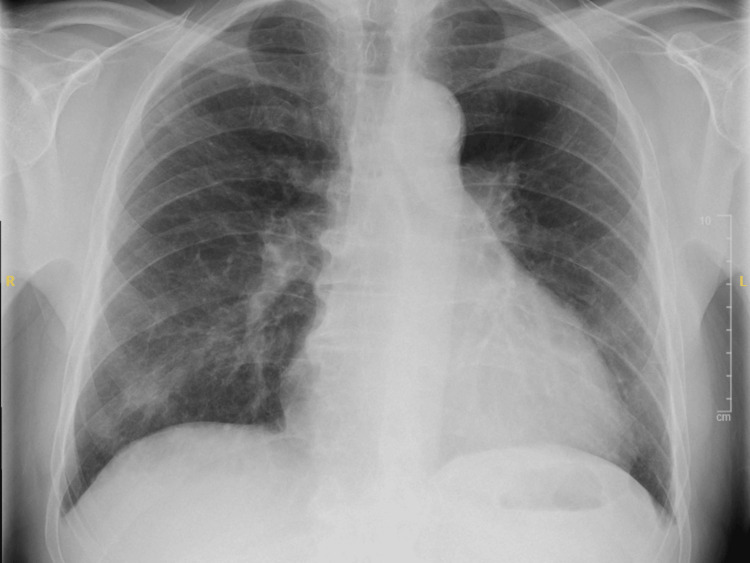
Chest X-ray showing an enlarged heart with a cardiothoracic ratio of 54%. Minimal bilateral pleural effusion and patchy air space opacification in the right lower zone, indicating an underlying infection, can also be seen in this X-ray.
